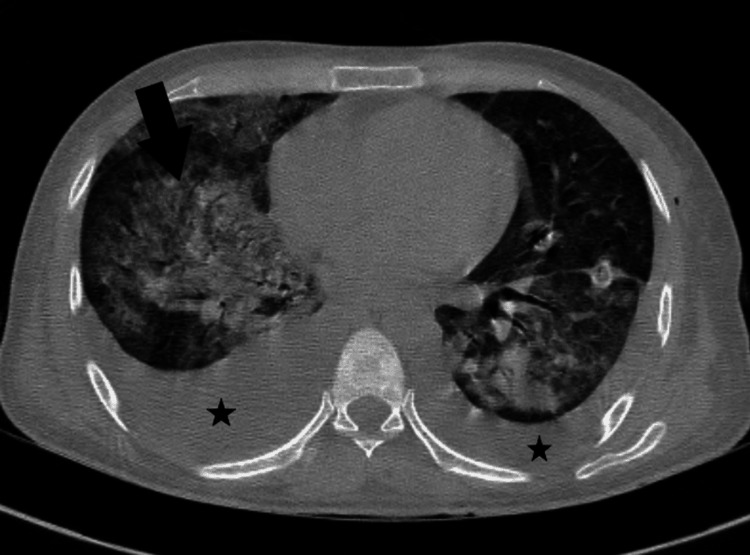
Non-contrast CT thorax axial image showing bilateral pleural effusion (star), right more than left, with collapse consolidation of underlying lung parenchyma. An arrowhead indicates diffuse ground glass opacities.
